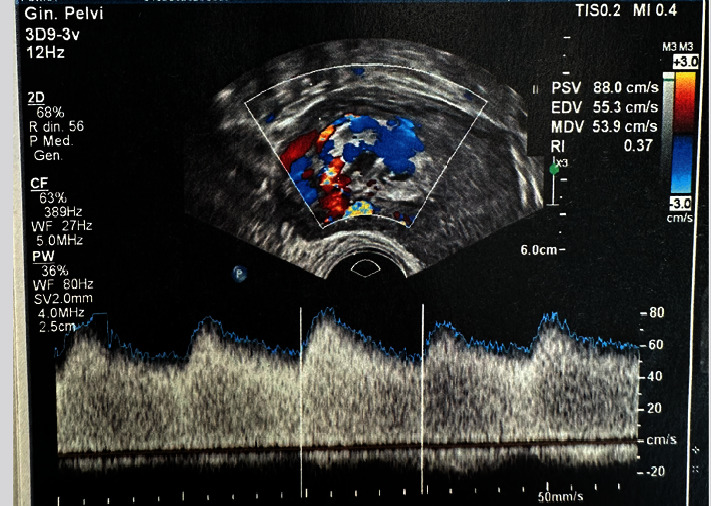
Transvaginal ultrasound with evidence of a nonhomogeneous mosaic area of the anterofundal wall with vascularization Colorscore 4 and PSV 88 cm/s (diagnosis of uterine arteriovenous malformation).
